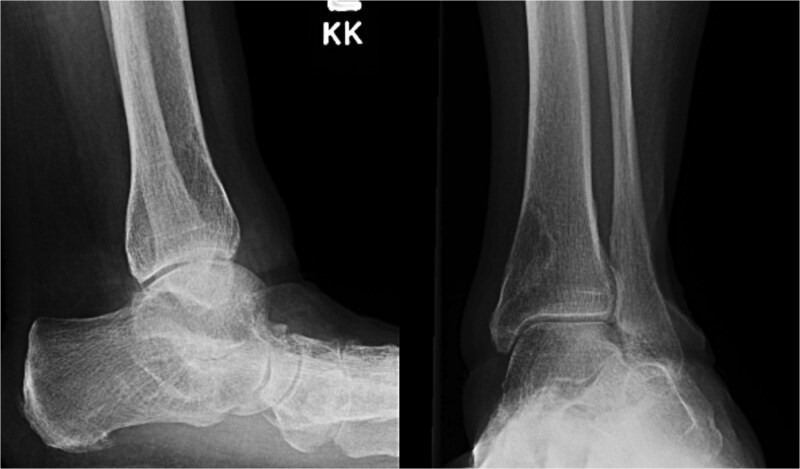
Preoperative anteroposterior and lateral radiographs of the left tibia and fibula demonstrating a spiral fracture of the distal tibial shaft with an associated proximal fibular fracture.
